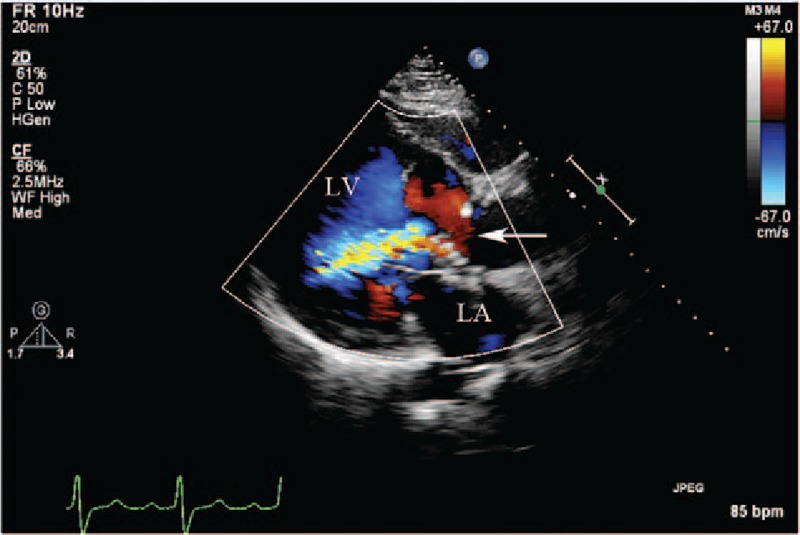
(Patient #11) Transthoracic echocardiogram (parasternal long axis view with color) showing the communication (arrow) from the right sinus of Valsalva aneurysm into the interventricular septal cavity, and the entry site of rupture from the false interventricular septal dissecting cavity into the left ventricular cavity. LA = left atrium, LV = left ventricle.
